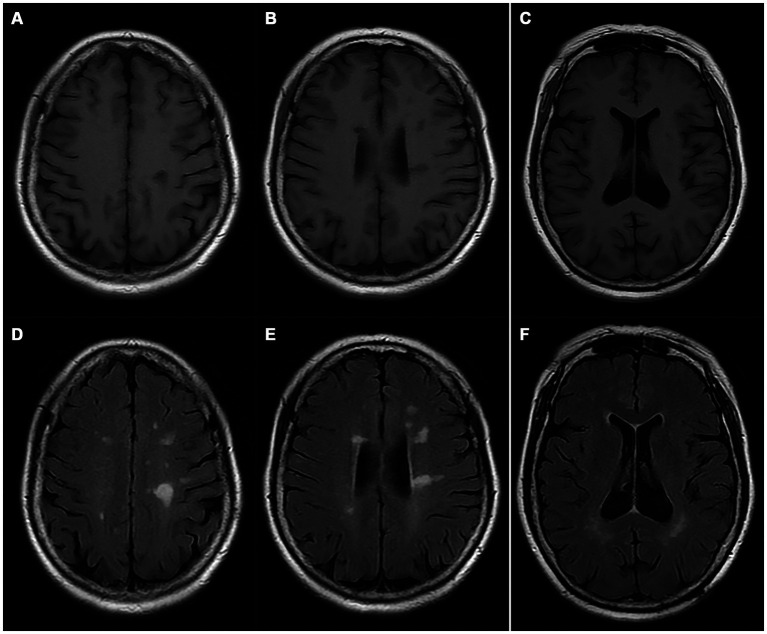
Magnetic resonance imaging of the proband (I-2). (A–C) T1WI; (D–F) T2 FLAIR images.
